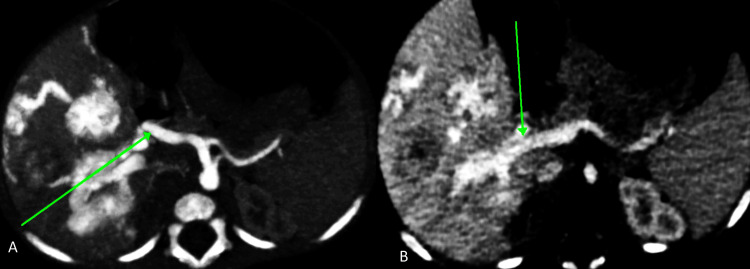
Axial sections of contrast-enhanced CT images showing (A) common hepatic artery (green arrow) and its branches appear dilated and torturous supplying lesions mentioned above; and (B) opacified portal vein (green arrow) and its right and left branches in the arterial phase-suggestive of arterioportal shunting CT: computed tomography
